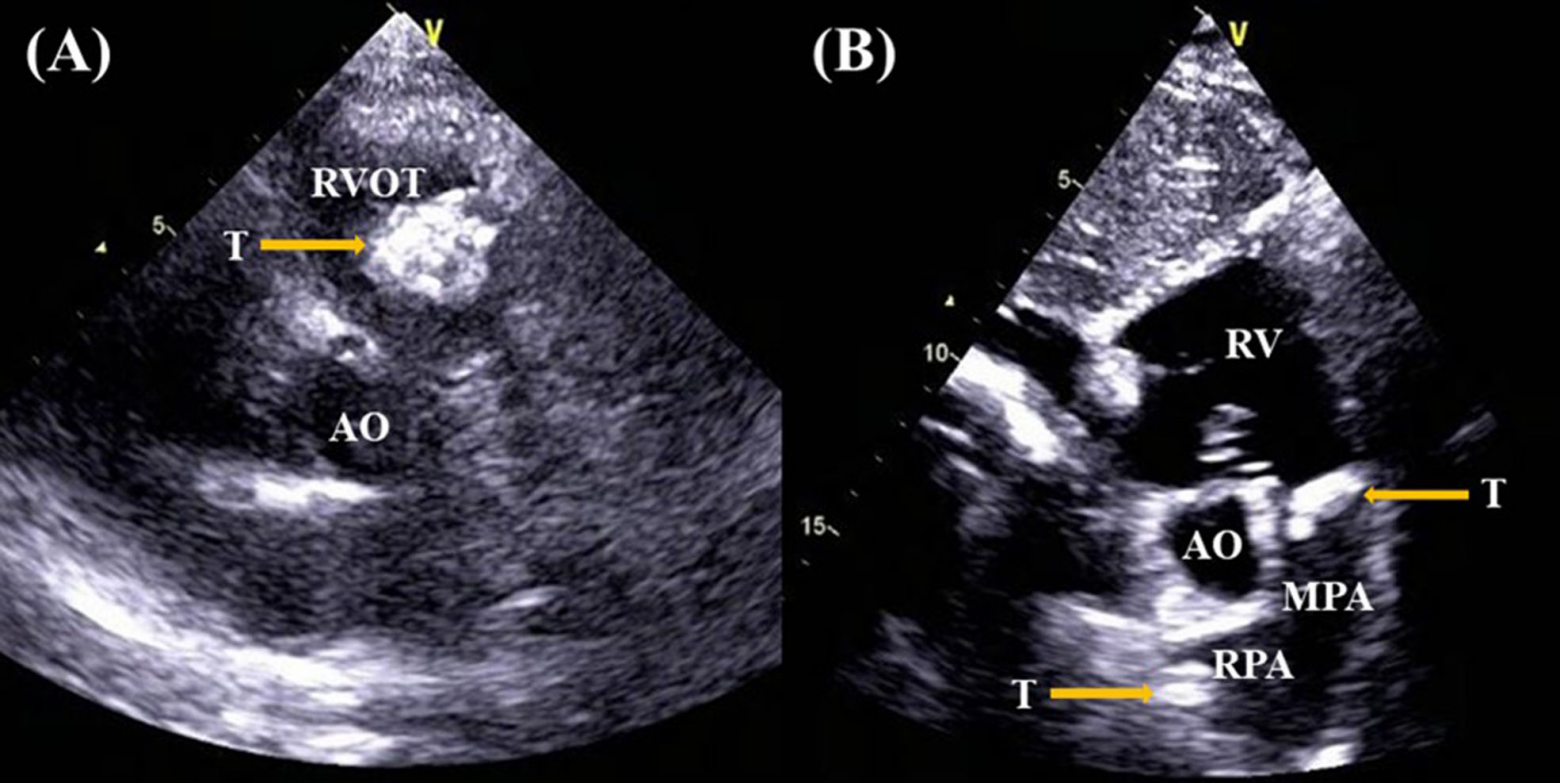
Transthoracic echocardiography revealed the presence of a mobile, polylobate shaped mass with mid-to-high echogenicity in the RVOT (A), another lesion in the RPA (B).
